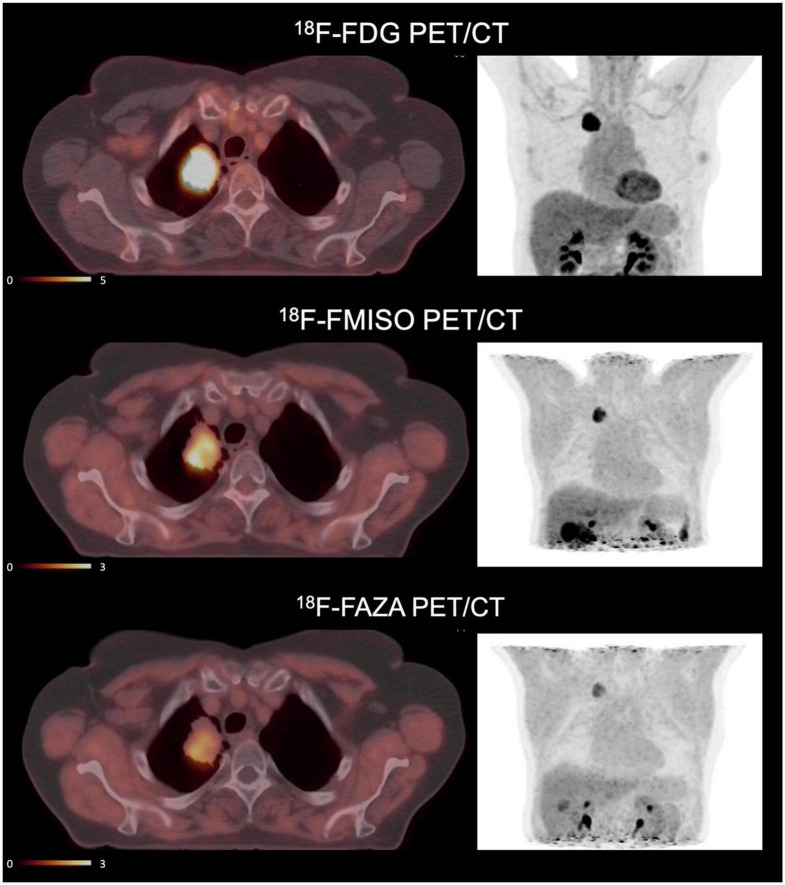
Example of PET imaging of a patient with [^18^F]-FDG, [^18^F]-FMISO and [^18^F]-FAZA. This patient with non-small cell lung cancer is included in a clinical trial “RTEP6” and signed an informed consent. Hypermetabolic regions are visualized by [^18^F]-FDG and hypoxic regions by [^18^F]-FMISO and [^18^F]-FAZA. Standardized uptake values (SUV) of [^18^F]-FDG are of an order of magnitude 5 times higher than SUV of [^18^F]-FMISO and [^18^F]-FAZA. The accumulation of tracers does not necessarily occur in the same tissue territories. The uptake of [^18^F]-FAZA is lower than [^18^F]-FMISO (33).
